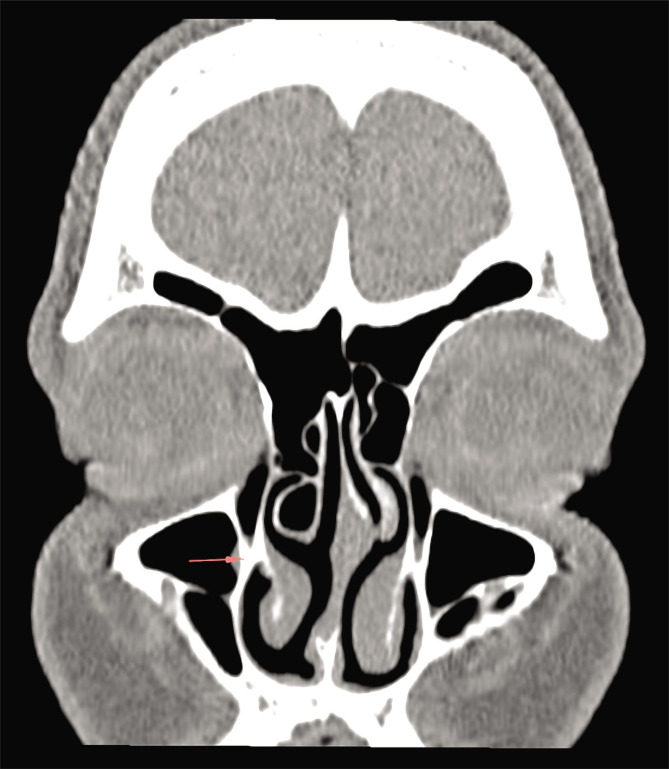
Coronal plane CT scan showing the insertion of the inferior turbinate on the frontal process of the maxillary bone.
